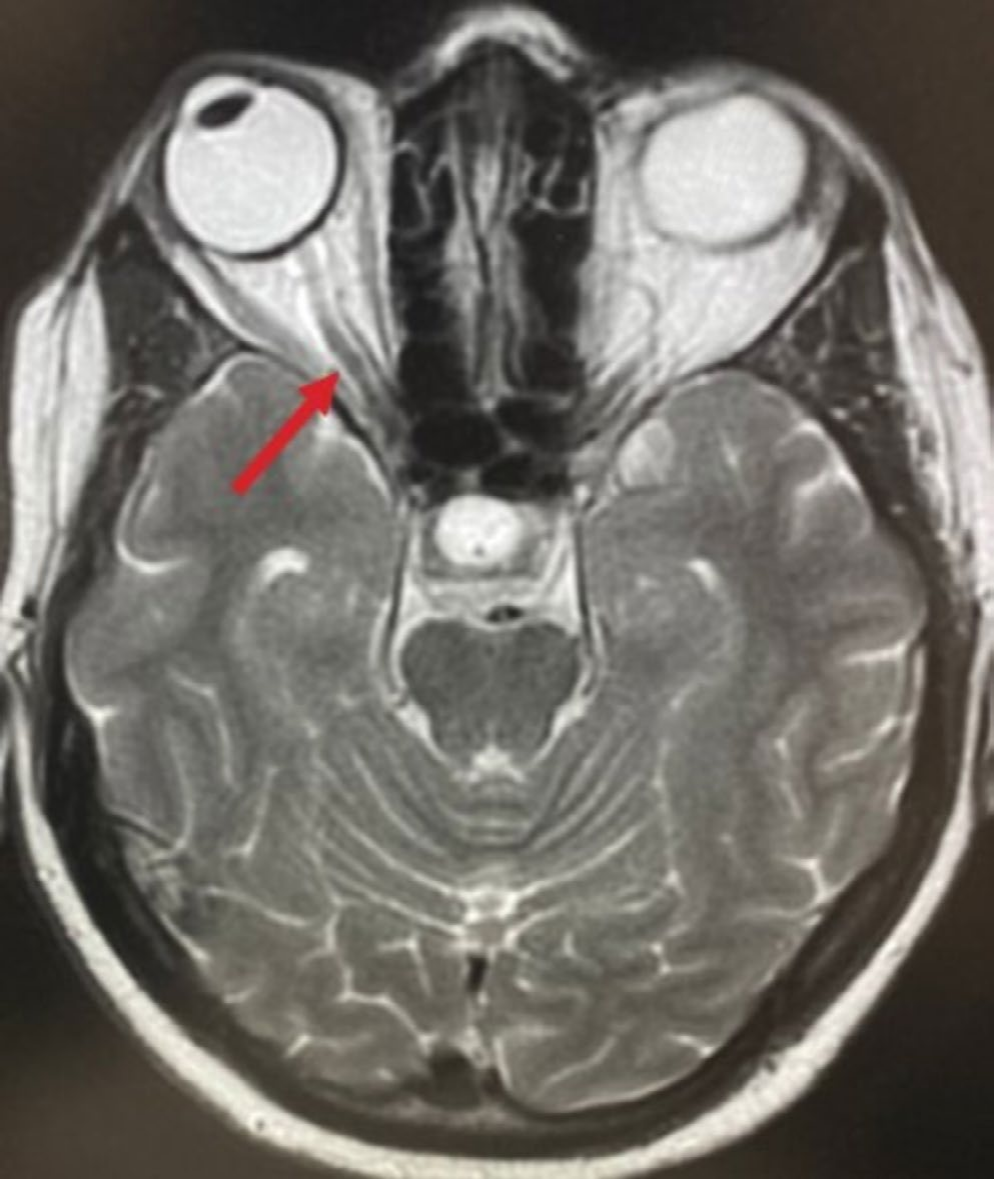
Shows a brain MRI image revealing with the red arrow pointing to the prominent perioptic subarachnoid space. This area contain CSF, and an enlargement may indicate elevated intracerebral pressure.
